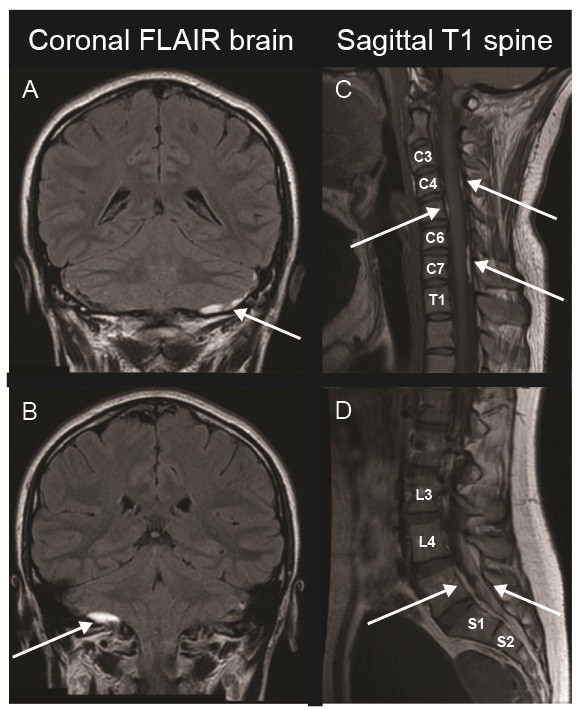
Diffuse spontaneous subdural hematoma associated with acquired hemophilia A. MRI showing coronal brain FLAIR sequence (A and B), sagittal T1 sequence of the cervical spine (C) and sagittal T1 sequence of the lumbar spine (D) demonstrating multiple subdural hematomas indicated by arrows. The patient had subdural hematomas located in the bilateral infracerebellar, cervical (C1-C7, maximal at C6-7), and lumbar (L4-S1) regions.
